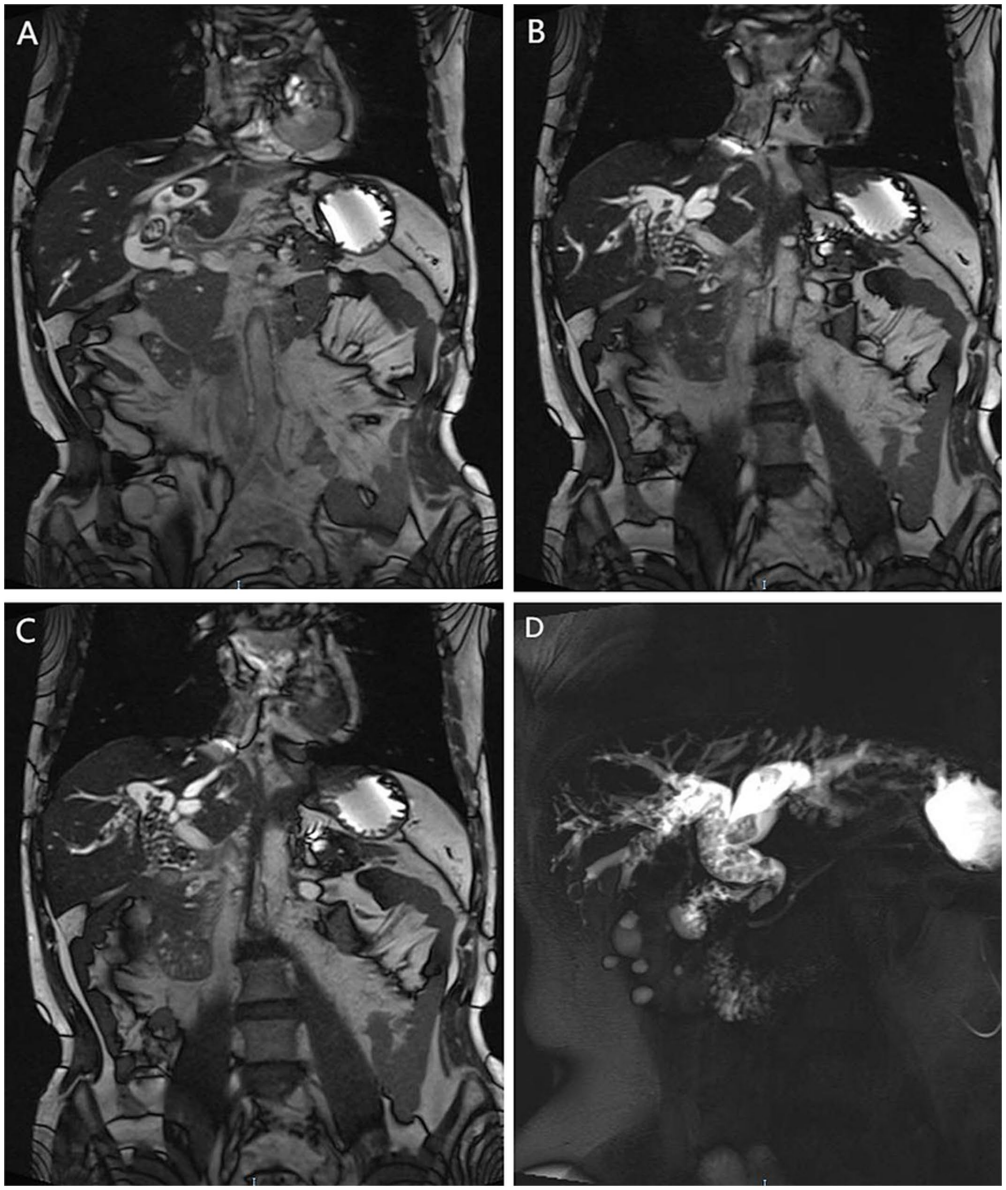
Biliary stone distribution visualized by MR/MRCP. (A) Coronal MR image: Stones in the left hepatic duct; (B,C) Coronal MR images: Stones in the right hepatic duct and common bile duct; (D) MRCP image: Overall morphology of the biliary tract and stone distribution.
